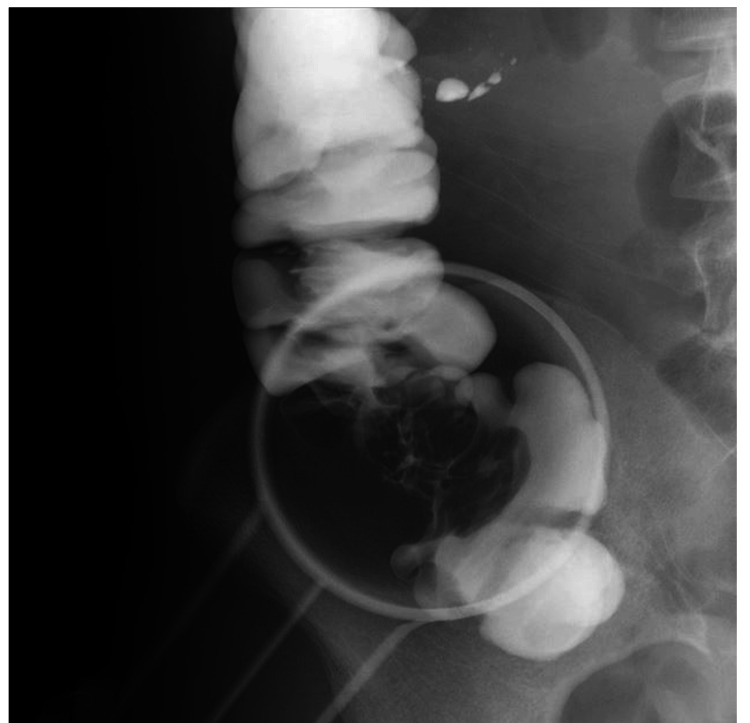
Gastrointestinal Gastrografin radiography of the ascending colon A filling defect in the ascending colon was observed on gastrointestinal Gastrografin radiography.
